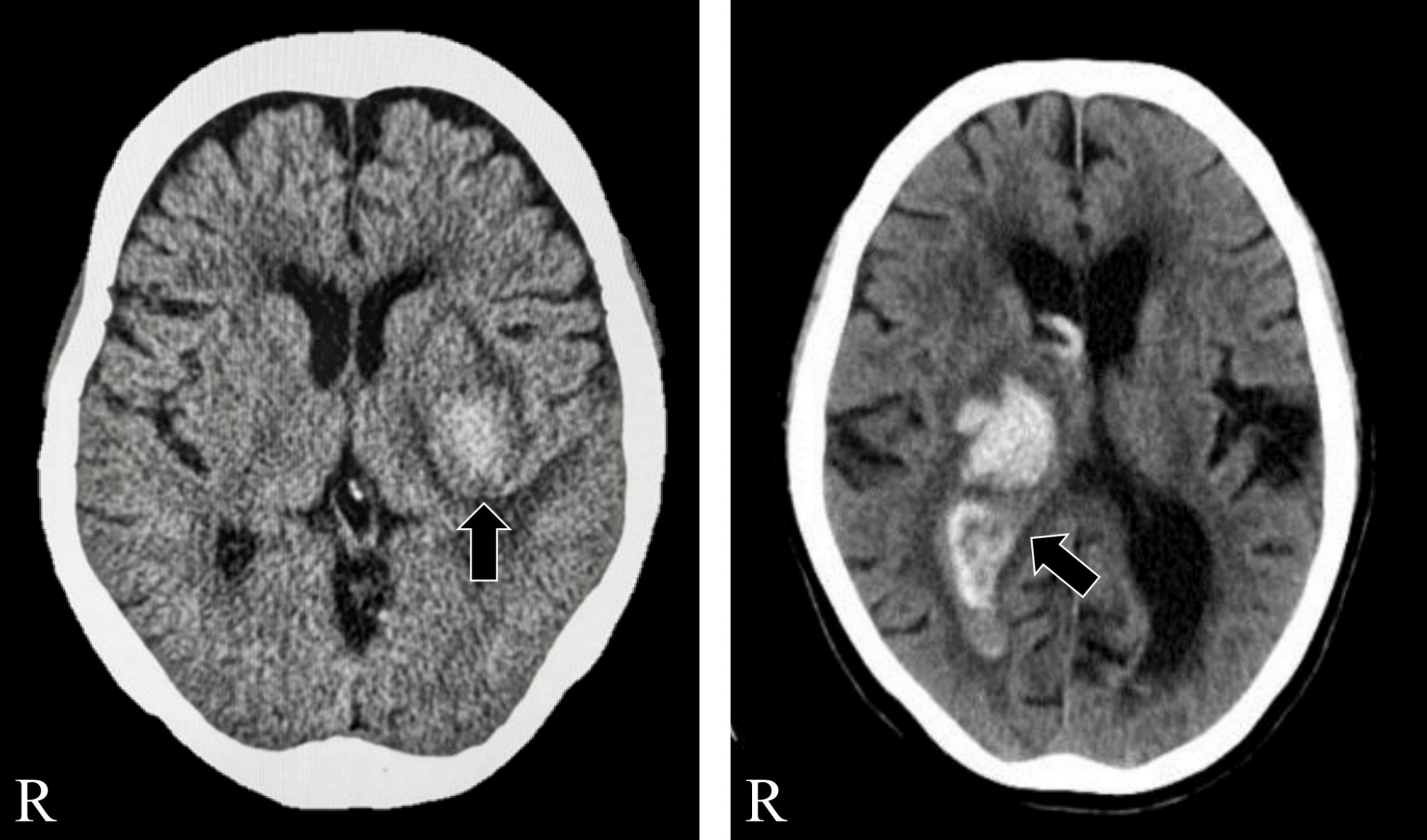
Computed tomography of the patients. Computed tomography of case 1 on the left and case 2 on the right.
